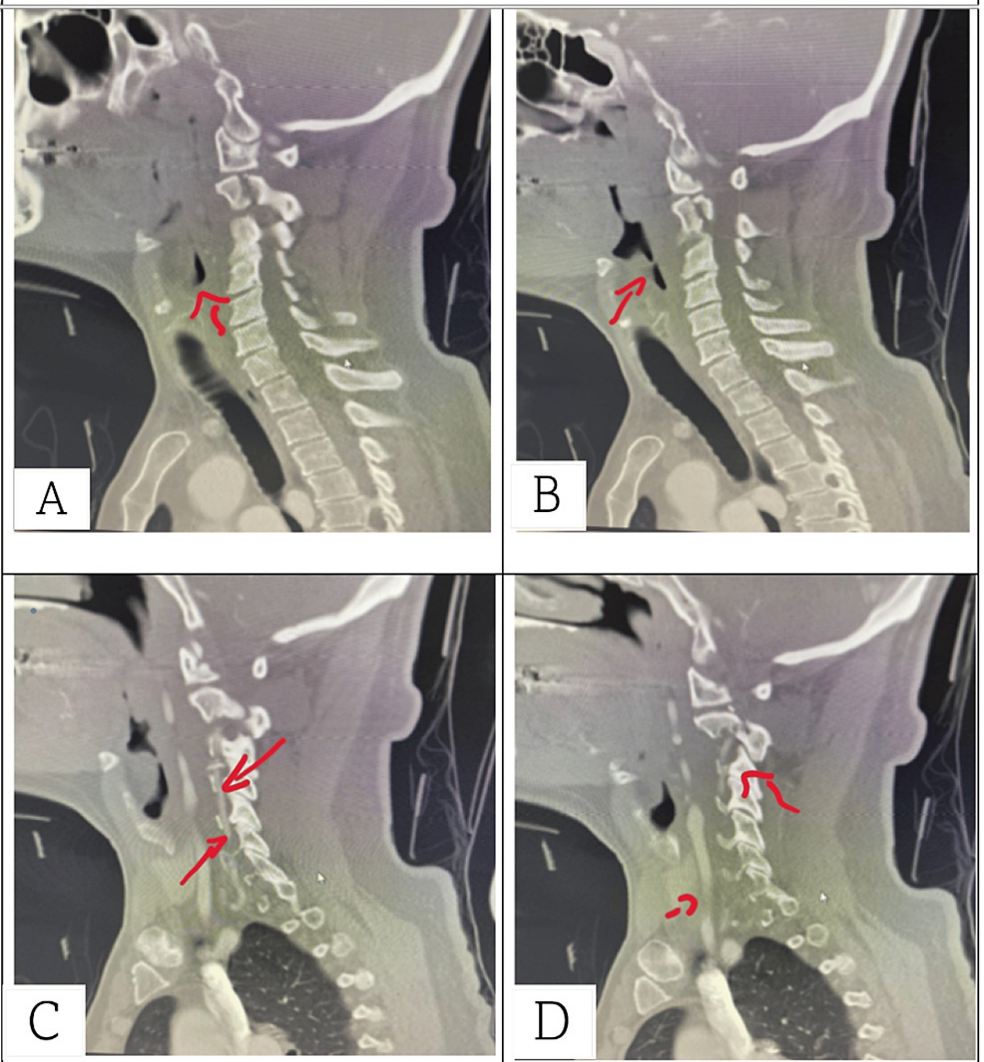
CT arteriogram (sagittal view) of the vertebral arteries with different sections labeled A, B, C, and D. The progression of the photos goes from internal to external. Image (A) shows the cervical spinal processes and the esophagus. Note the hypoechoic portion above the esophagus, indicating the left lateral side of the oropharynx. Image (B) shows slight external progression from the spinal cord where we see more of the oropharynx. This is to indicate the progression of CT going more lateral. Image (C) is more lateral, on the patient's right side. The hyperechoic portion, where we see the contrast, along the spinous processes is the vertebral artery. More anterior we see contrast uptake in the carotid artery. Note the lack of contrast outside of the spinal processes, indicating no perforation of the artery. Image (D) shows a more lateral view of the patient's neck. Note the contrast attenuation in the vertebral artery as well as no dispersion of the media.
